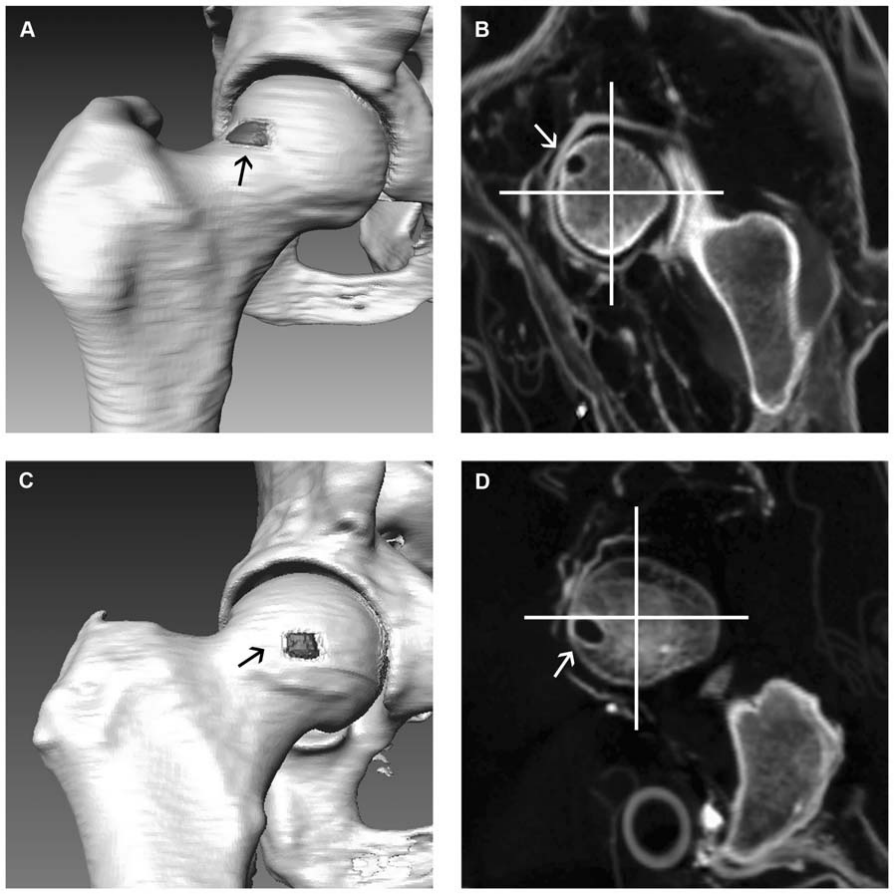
Location of HPs. Typical location of HPs in the superior portion of the anterior femoral neck (A, B) and less common location in the transition zone between the superior and inferior portion of the anterior femoral neck (C, D). (A, C) Modified three-dimensional CT reconstructions of living patients with HPs. (B, D) Parasagittal (perpendicular to the femoral neck axis) CT reformation images of case 2 and 3 with division into a superior (between 9 and 12 o'clock) and inferior (between 6 and 9 o'clock) portion.
